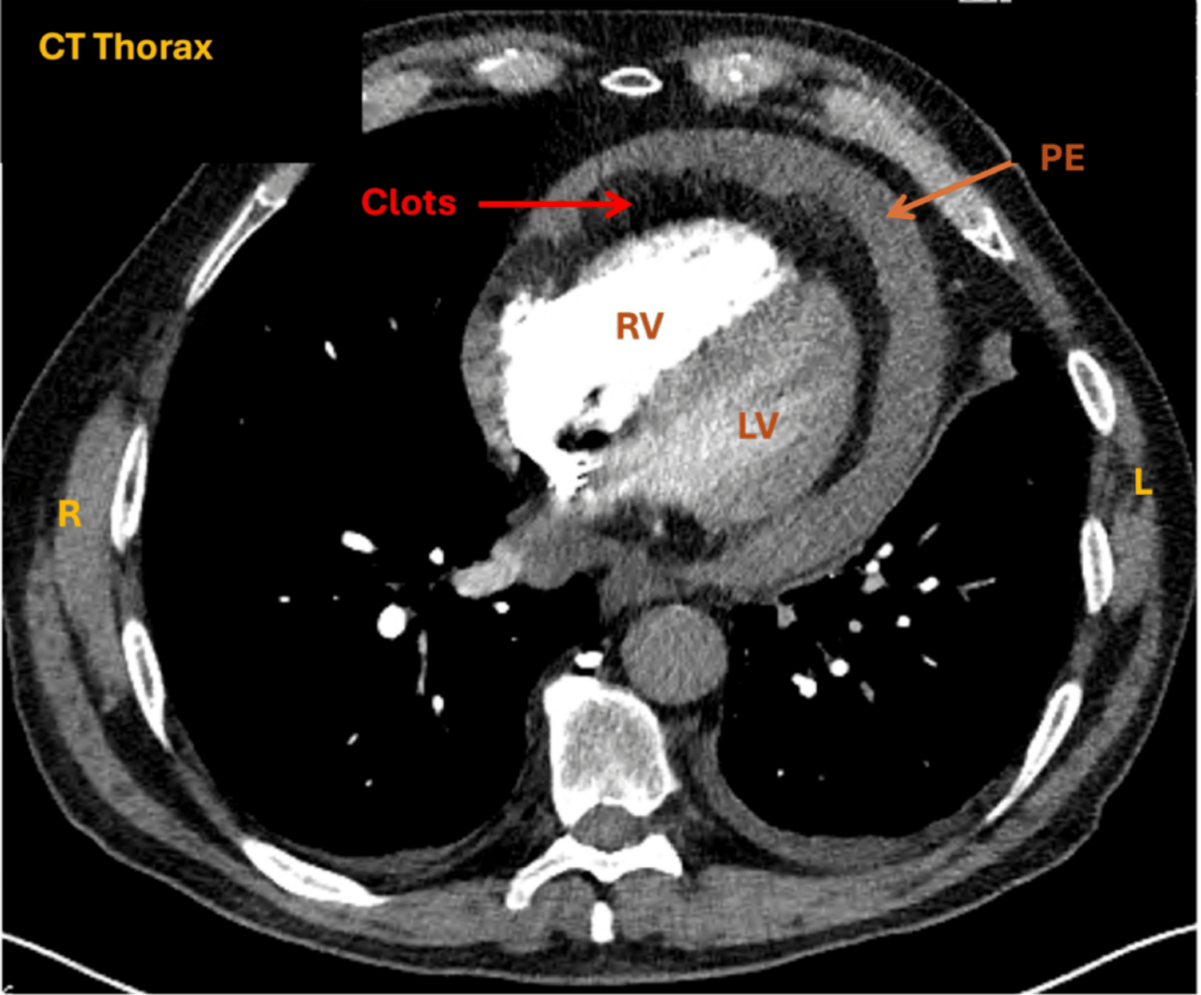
Contrast CT thorax demonstrating presence of pericardial effusion (orange arrow) CT: computed tomography, PE: pericardial effusions, LV: left ventricle, RV: right ventricle This image has not been published elsewhere.
